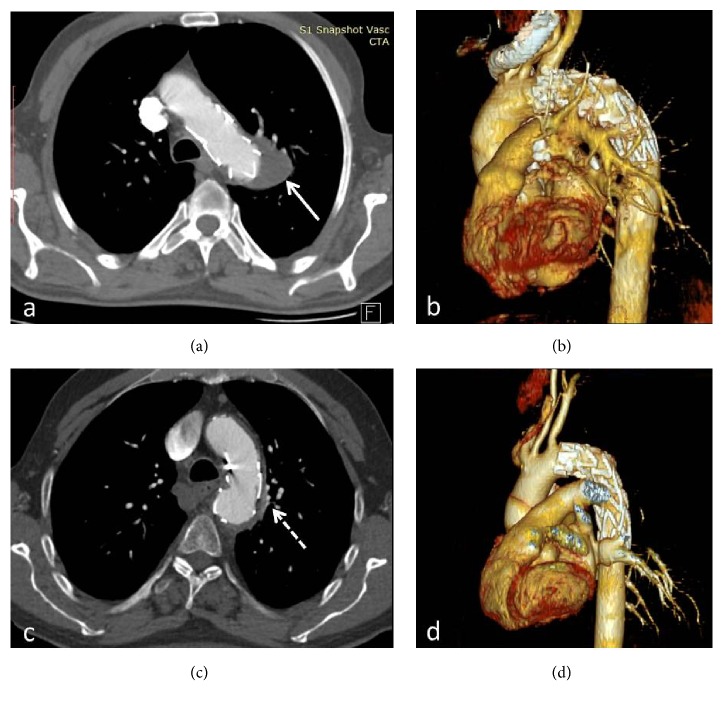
Case 1 (a, b). Case 2 (c, d). Contrast-enhanced CT and VRT images show complete occlusion of the aneurysm with thrombosis and shrinkage, especially in case 2 (white arrow in (a), dashed arrow in (d)).
